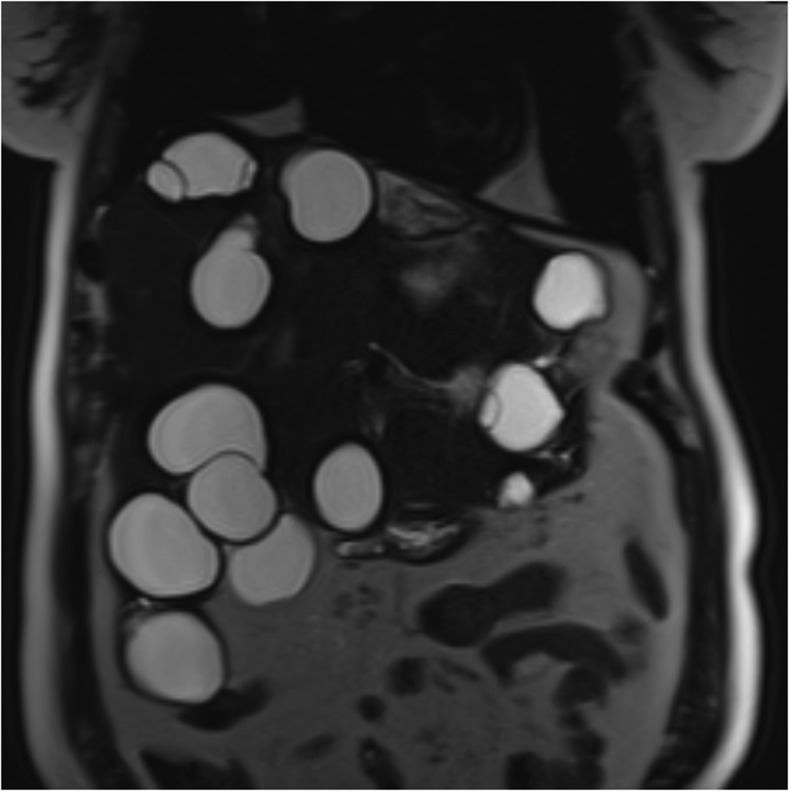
Coronal view of a T2-weighted magnetic resonance imaging (MRI) demonstrating multiple hydatid cysts of the liver.
